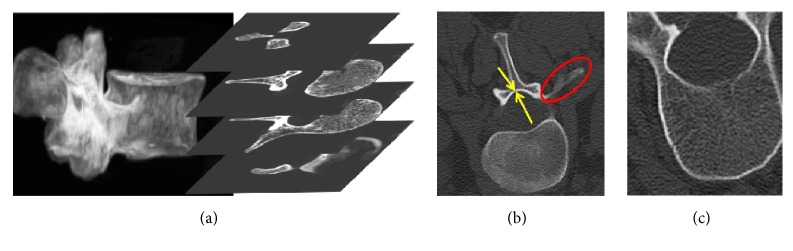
Challenges in segmentation of lumbar vertebrae CT images: (a) topological variation of vertebrae anatomical structure, (b) irregular boundaries (double boundary and weak boundary), (c) image noise.
